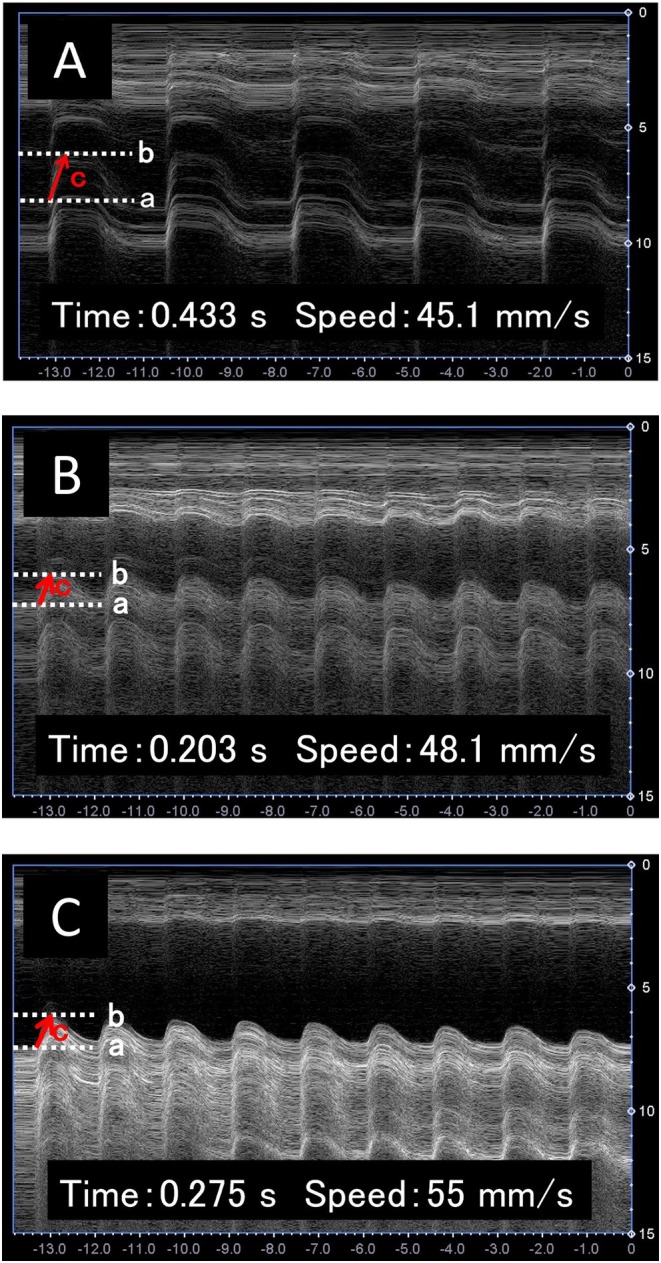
Alterations in bladder‐base elevation waveform are observed through M‐mode ultrasonography during pelvic floor muscle training (PFMT). a: Baseline of the bladder base; b: Maximum elevation line; c: Displacement used for quantitative measurements. (A) During PT intervention (bladder base elevation time, 0.433 s; bladder base elevation speed: 45.1 mm/s). (B). After four months of continued PFMT (bladder base elevation time, 0.203 s; bladder base elevation speed: 48.1 mm/s). (C) After vaginal pessary removal (bladder base elevation time, 0.275 s; bladder base elevation speed, 55 mm/s).
